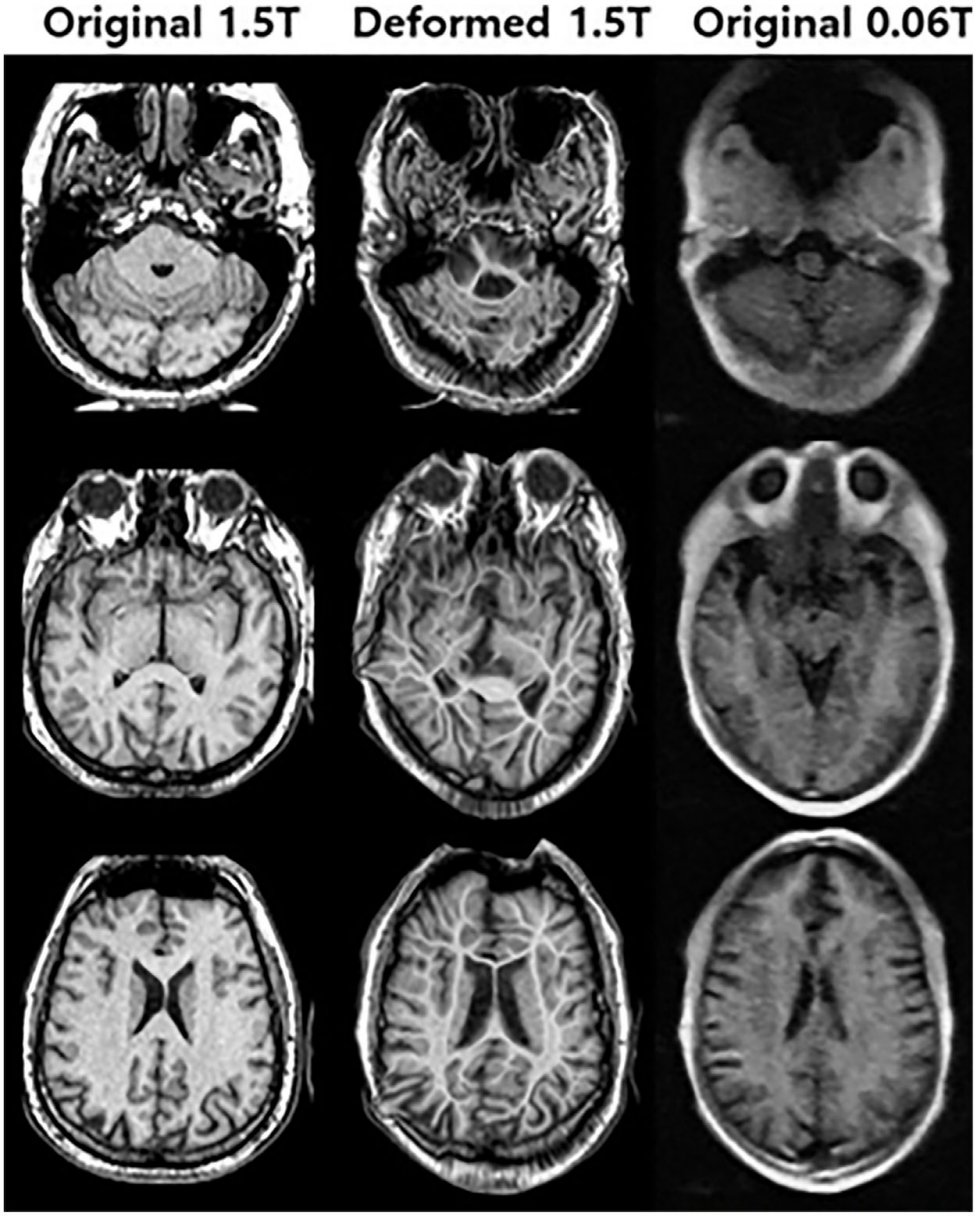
Deformed 1.5 Tesla MR image from original 1.5 Tesla MR image according to 0.06 Tesla MR image in step 1 (deformable registration).
